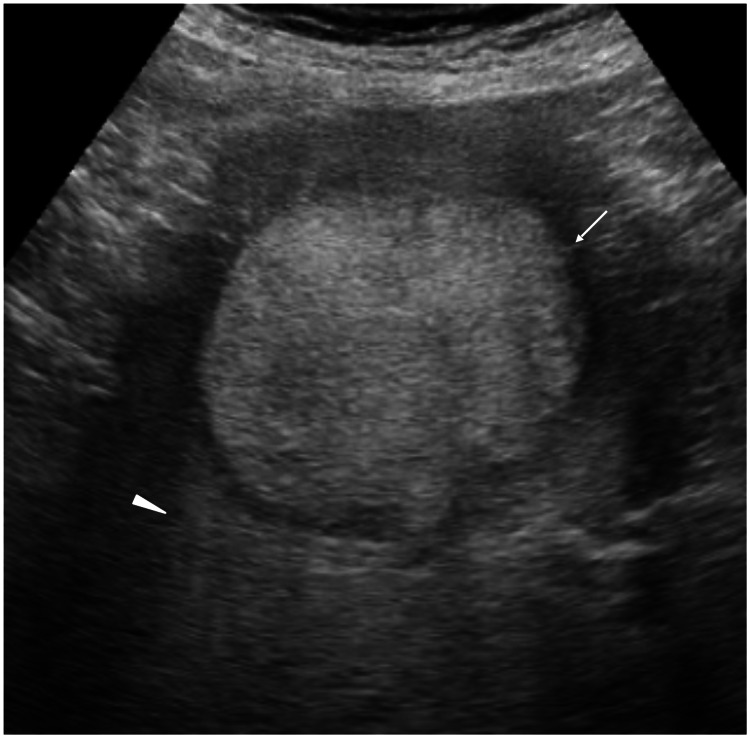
Ultrasound findings Ultrasound showed an oval mass with very high internal echoes, enhanced posterior echoes (arrowhead), and a presumed fibrous capsule (arrow).
